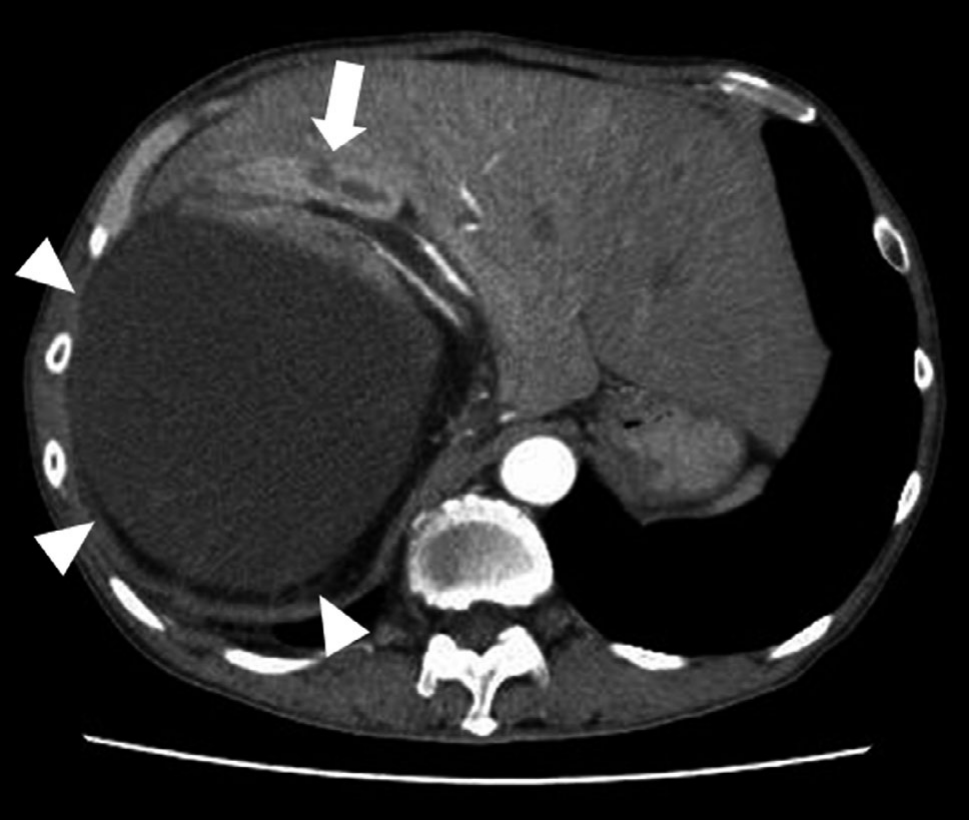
Abdominal CT scan showing the homogeneous enhancement of the tumor measuring >20 cm (white triangle), which compressed the right kidney (white arrow).
